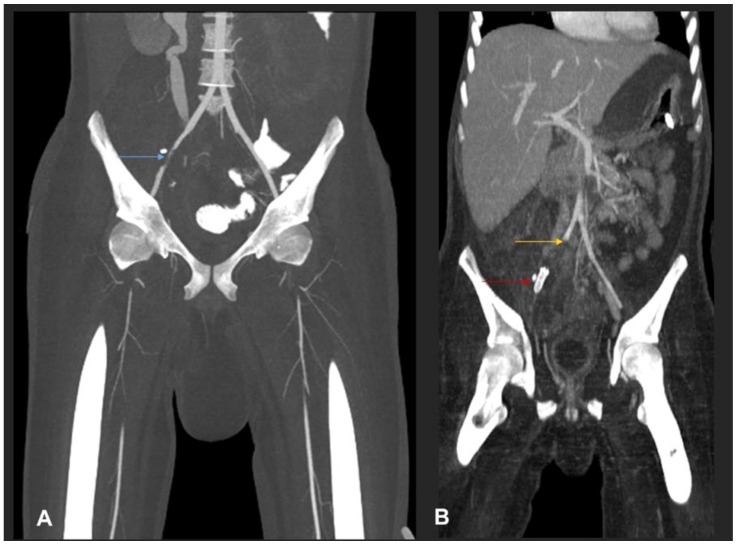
(A) MIP coronal CT angiography of aorta and lower limb shows a segmental narrowing and luminal irregularities of right external iliac artery (blue arrow). No occlusion. Distal run off was satisfactory. Rest of the lower limb vessels were patent and normal (not shown). (B) MIP coronal image of the aorta and lower limbs shows cut-off of the proximal right common iliac artery suggestive of thrombotic occlusion (yellow arrow). A stent is seen in the right external iliac artery (red arrow). Right iliac fossa shows extensive inflammatory phlegmonous mass-like soft tissue thickening.
